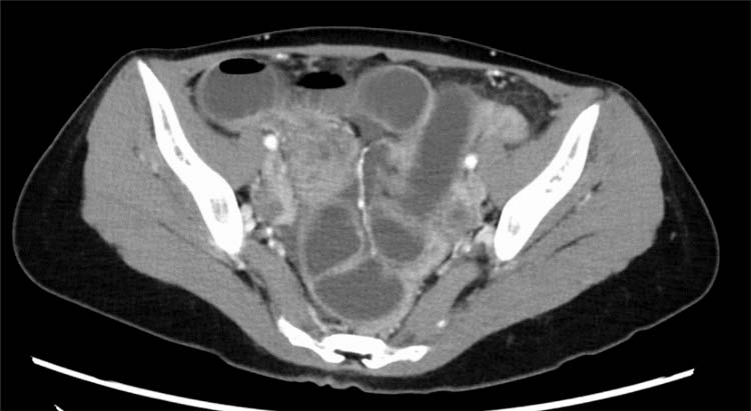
Abdomino-pelvic CT image; dilatation of the small bowel with transition point at the terminal ileum.
